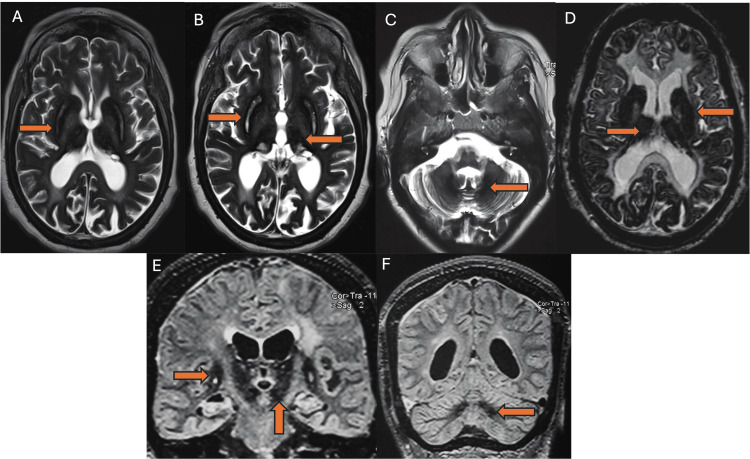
Brain MRI showing bilateral and symmetrical hyposignal in the striatum, globus pallidus, thalami, substantia nigra, and dentate nuclei apparent on axial T2- (A, B, C); SWI- (D) weighted sequences and coronal FLAIR sequences (E, F) SWI: susceptibility-weighted imaging; FLAIR: fluid-attenuated inversion recovery
